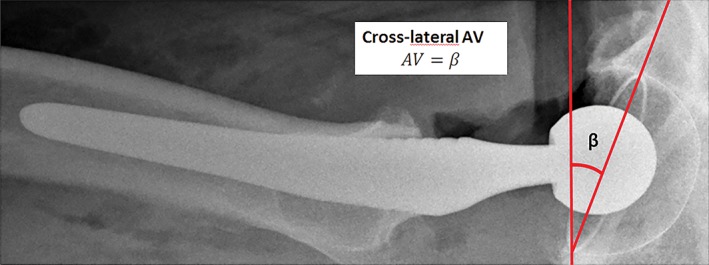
Category 2 method. Definition and algorithm of the included anteversion measurements on a cross‐lateral radiograph^16^. The angle is measured by the opening of the cup in relation to the axis of the respective plane. AV, anteversion; β, angle.
